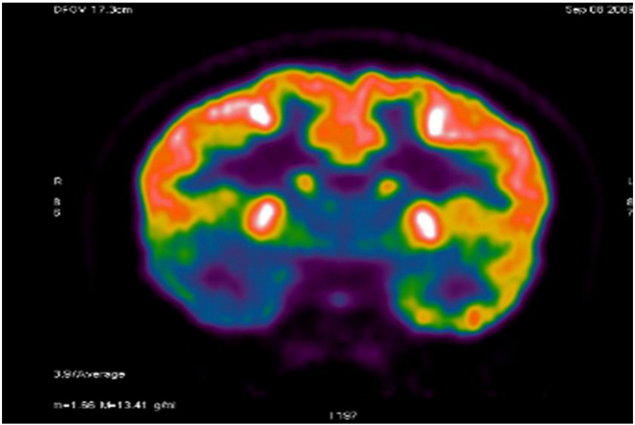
The PET study demonstrates decreased FDG metabolism involving the right temporal lobe.
